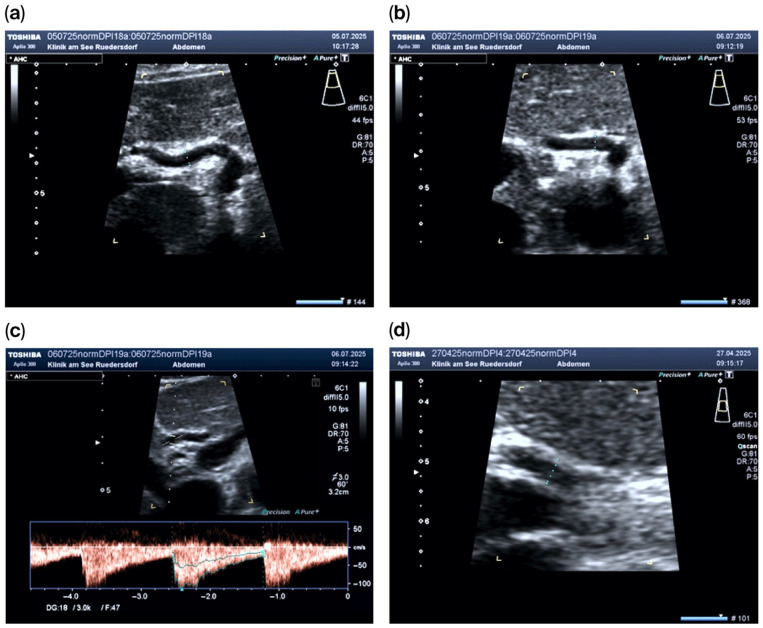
Doppler sonographic approaches to the common and proper hepatic artery. (a) Sinuous course of the common hepatic artery. (b) Transverse course of the common hepatic artery. (c) Doppler assessment of the proper hepatic artery. (d) Determination of the proper hepatic artery diameter. Note. A favorable vessel course of the common hepatic artery allows a feasible insonation angle (a), whereas a transverse course precludes an insonation angle ≤ 60° despite adequate B-mode visualization (b). The proper hepatic artery is assessed via a transverse upper abdominal approach (c). Due to its small diameter (typically <4 mm), accurate measurement requires high-resolution magnified imaging and application of the leading-edge method (d).
